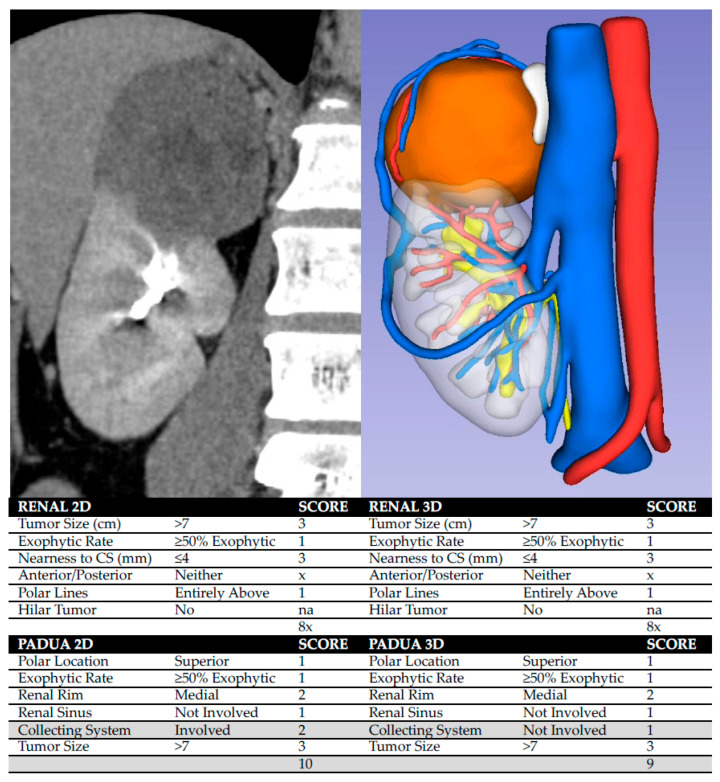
Comparison between a 2D CT urographic coronal image and an HA3D model. (Left panel): Conventional 2D CT imaging used for RENAL and PADUA score calculation, in which the collecting system (CS) appears to be involved. (Right panel): HA3D model showing a volumetric representation of the renal tumor, renal parenchyma, arterial and venous anatomy, and a non-involved collecting system. In this case, HA3D-based assessment allowed improved visualization of the CS, influencing PADUA score assignment and resulting in downgrading from a high- to a moderate-risk category.
